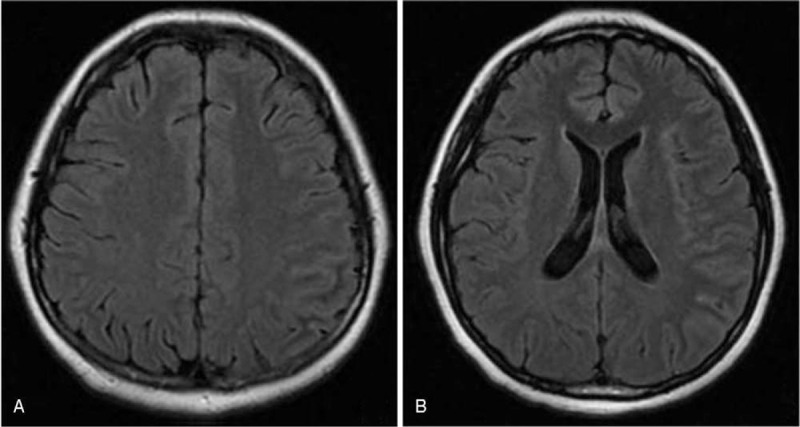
On day 14 after admission, axial T2-FLAIR images showed partial resolution of the left cerebral cortical lesions (A, B). T2-FLAIR = T2-weighted fluid-attenuated inversion recovery.
